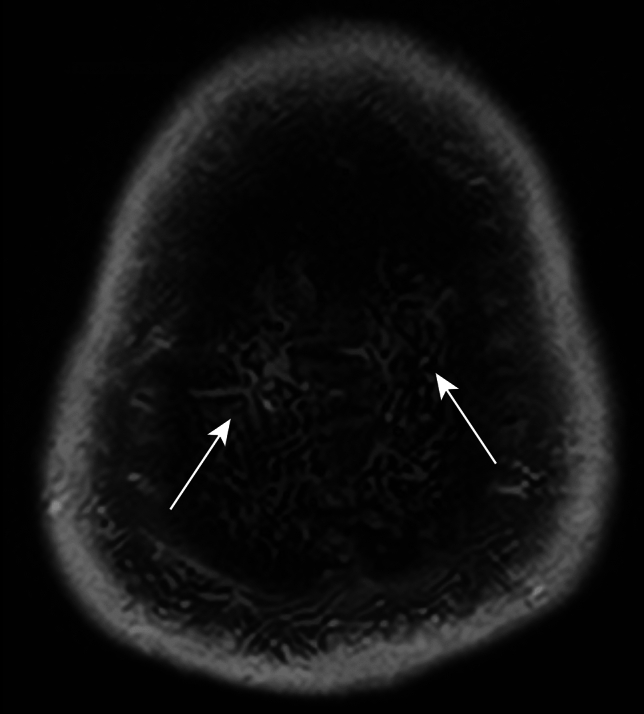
Reticular high-signal regions (*arrows*) exceeding the threshold on a contrast-enhanced motion-sensitized driven equilibrium (MSDE) T1-weighted transverse image, whose association with the diploic veins was uncertain
